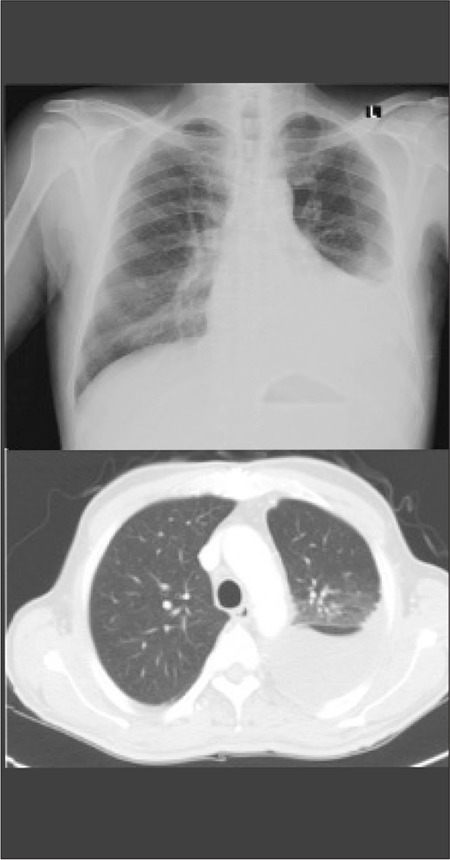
Chest X-ray and chest CT show a massive, left-sided pleural effusion.
